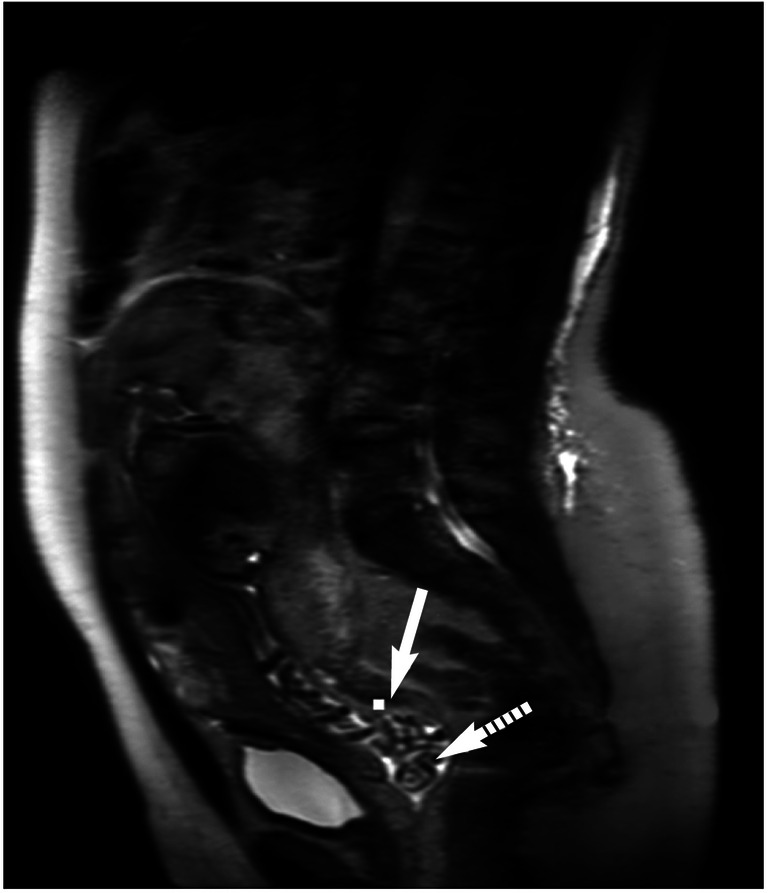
Cord prolapse in a 37-year-old woman imaged at 23^+5^ weeks’ gestation. Sagittal T2 turbo spin echo MR image acquired as part of a research study investigating the development of fetuses at high risk of preterm birth shows the umbilical cord prolapsing into the vagina (*dotted arrow*). The cervix is marked with a *solid arrow*
